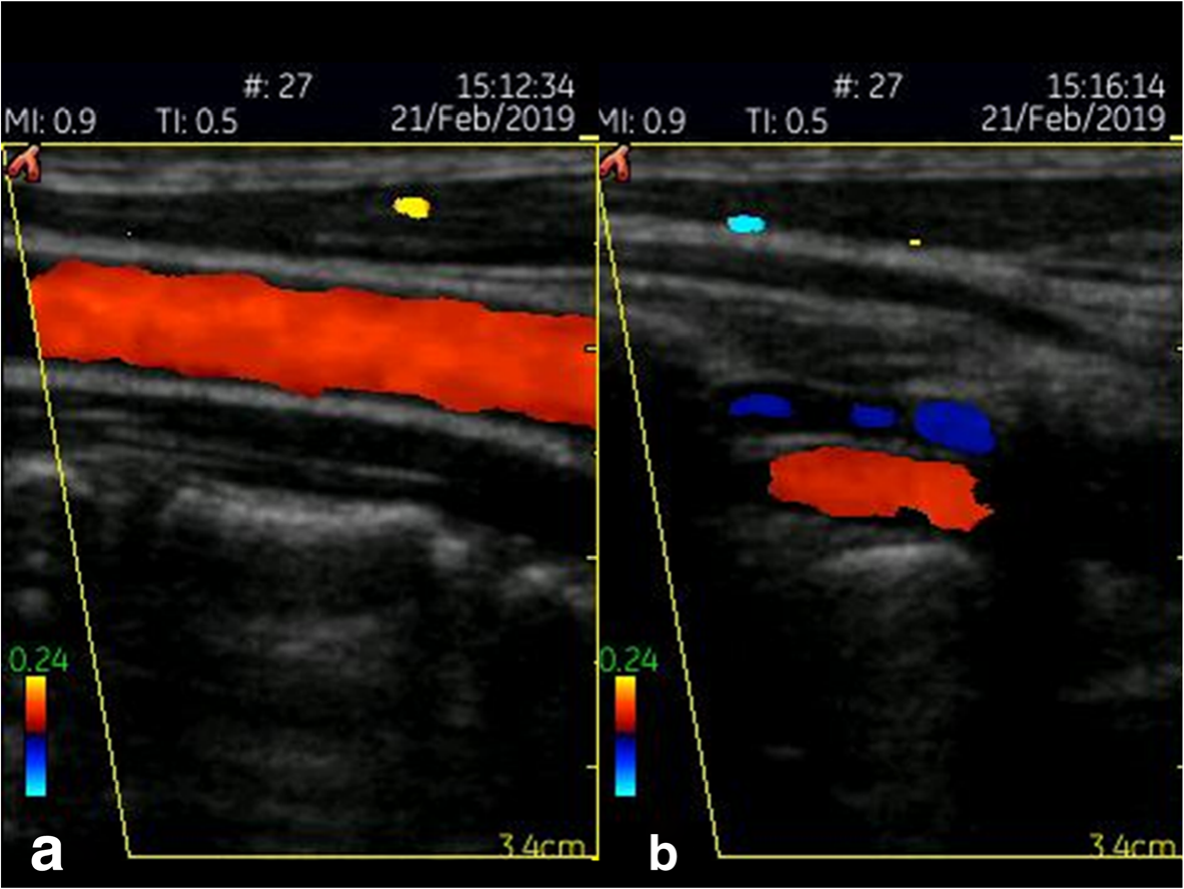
GE Healthcare VScan (linear array transducer): Colour Doppler image of the carotid (a) and vertebral artery (b)
